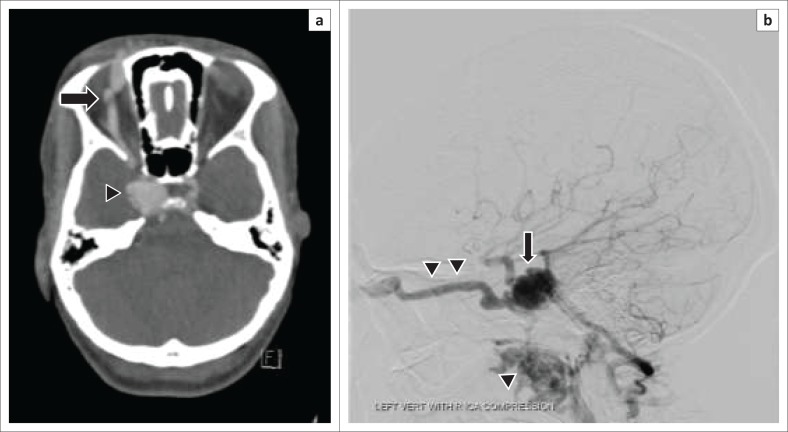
A 24-year-old female presented with a spontaneous history of proptosis, visual disturbance and pulsatile tinnitus: (a) Axial source image from a computed tomography angiography demonstrates early enhancement of a distended right cavernous sinus (arrowhead) and a dilated ipsilateral superior ophthalmic vein (arrow). (b) Lateral left vertebral artery angiogram with carotid compression (Heuber manoeuvre) in the same patient displays early filling of the distended cavernous sinus via the fistula (arrow) with drainage via anterior and inferior venous pathways (arrowheads).
